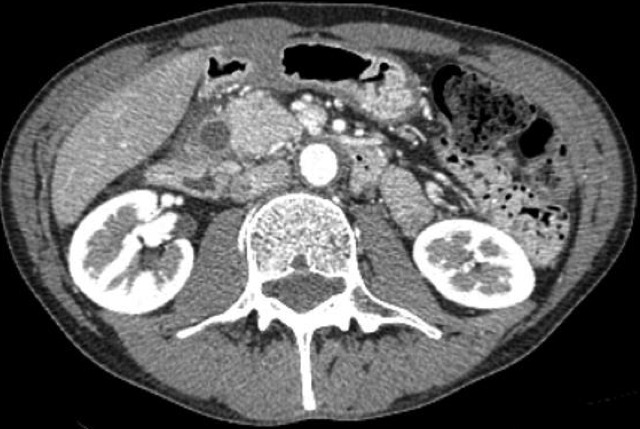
Abdominal CT image of the lesion in the head of pancreas
